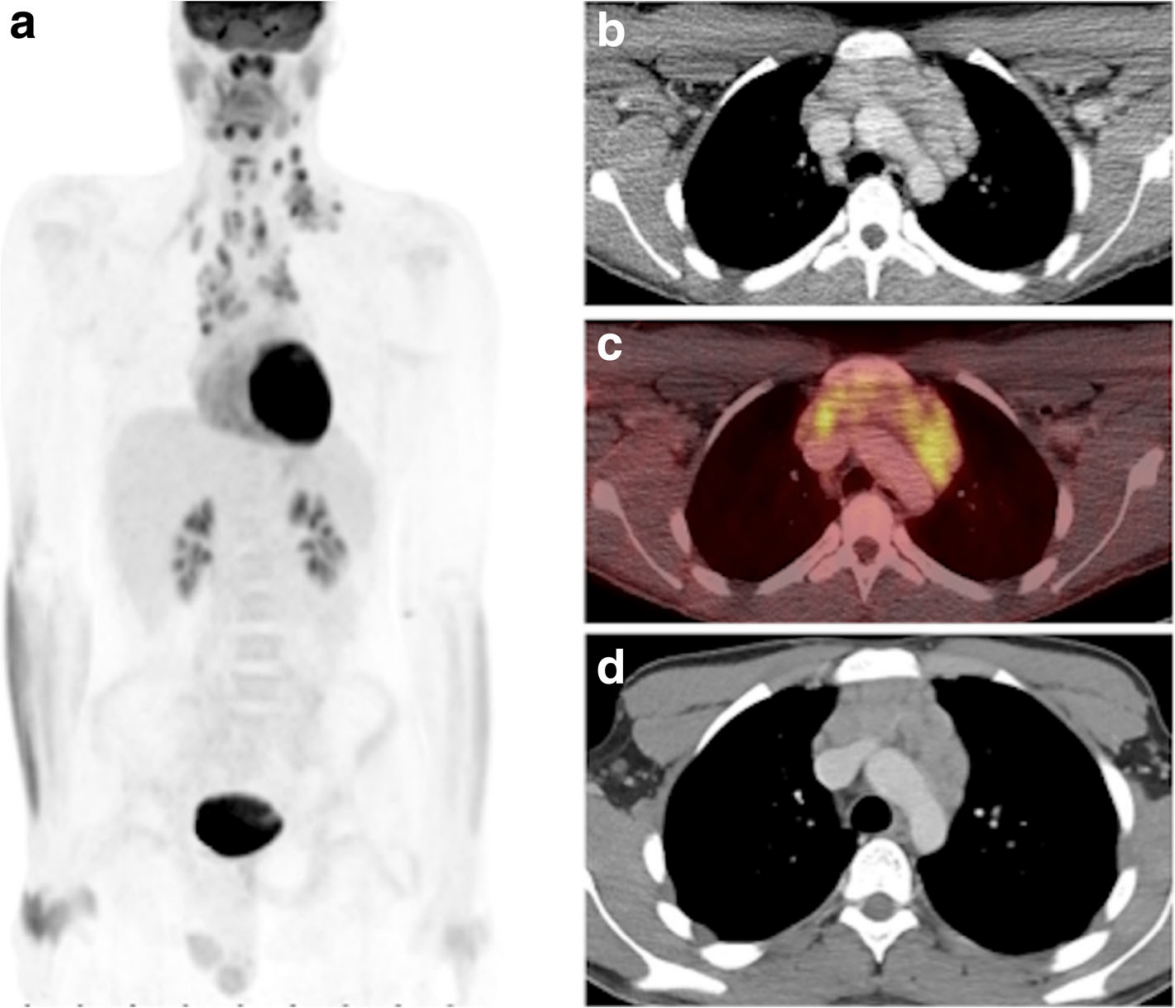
Contrast enhanced low dose attenuation correction CT from PET/CT exam compared to diagnostic CT. The low dose attenuation correction CT, performed with IV contrast (b, c) provides anatomic localization of the PET findings (a), and – while not of diagnostic quality – is comparable to the diagnostic CT (d), and has sufficient diagnostic information to warrant a thorough review
